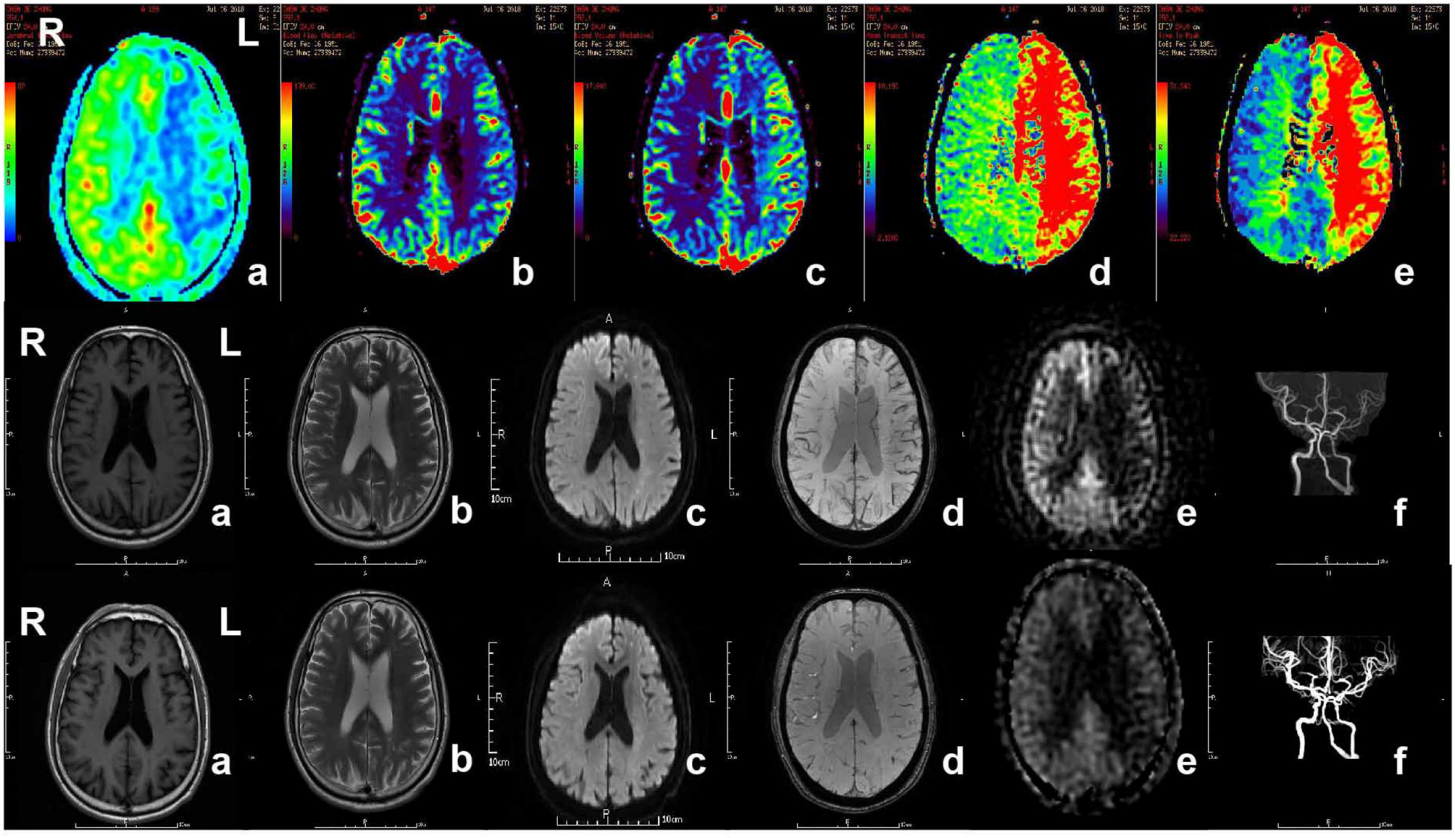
MR imaging of the patient with acute ischemic stroke. The patient, 71-year-old male, suffered from weakness of right limbs, alalia and was unable to walk 3 h prior to the imaging. Medical history included hypertension, diabetes and varicosity of both lower extremities. Physical examination showed right facial paralysis, the muscle strength of right limbs was grade I, right Babinski sign (+), Chaddock sigh (+), and NIHSS score was 13. The diagnosis of the patient is acute ischemic stroke. The patient was given multimodal MRI and MRA before and after recanalization. The first row showed perfusion-weighted imaging. a and b, Lower CBF in left cerebral hemisphere. c, Higher CBV in left cerebral hemisphere. d, Longer MTT in left cerebral hemisphere. e, Longer TTP in left cerebral hemisphere. The second row and third row showed MRI and MRA before recanalization and after recanalization, respectively. a, b, and c, In the left frontal lobe and lateral ventricle had sporadic dots with slightly longer T1 signal, longer T2 signal higher signal, respectively, in T1WI, T2WI, DWI. d, No cerebral microbleeds in SWI. e, Original ASL. f, MRA showed the intracranial segment of the left internal carotid artery and the left middle cerebral artery were significantly narrow in the second row, while recanalization got in left internal carotid artery and middle cerebral artery. MR, magnetic resonance; MRA, magnetic resonance angiography; SWI, susceptibility weighted imaging; ASL, arterial spin labeling; CBV, cerebral blood volume; CBF, cerebral blood flow; TTP, time to peak; MTT, mean transit time.
